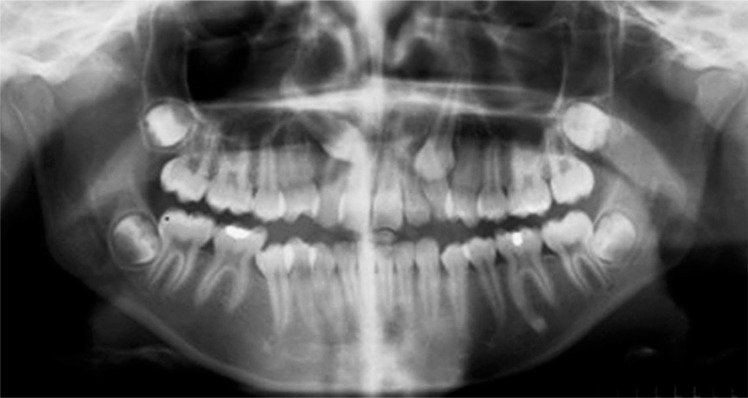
Scanned conventional panoramic radiograph.
